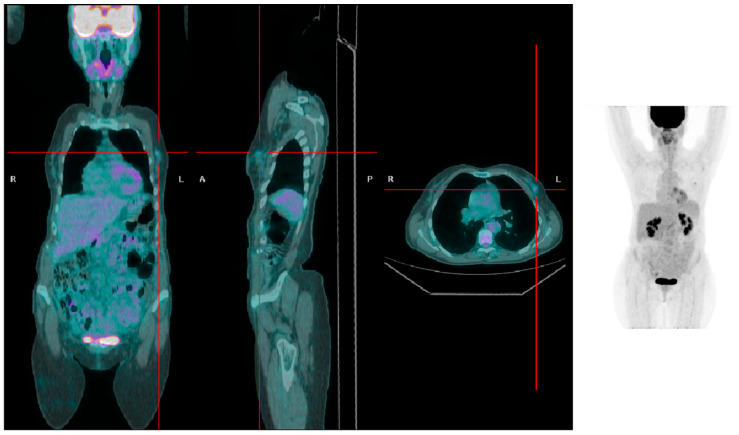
A patient with low BMI presenting with a left-sided breast tumor with mild [^18^F]FDG uptake (SUVmax 1.5).
